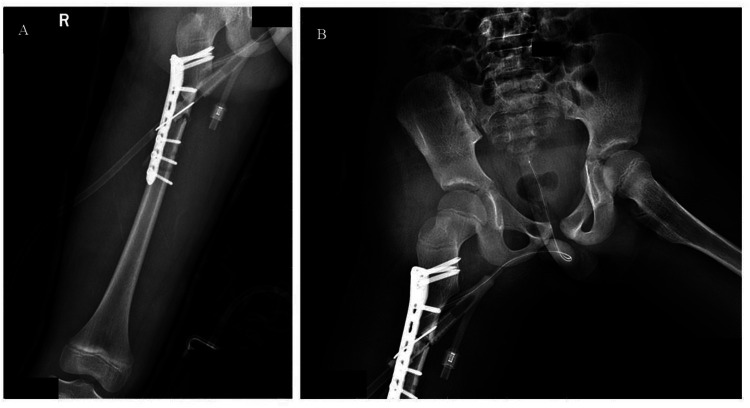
On postoperative day 3, radiograph demonstrates satisfactory alignment of the right femoral shaft fracture following internal fixation (A), with the femoral head well-seated (B).
